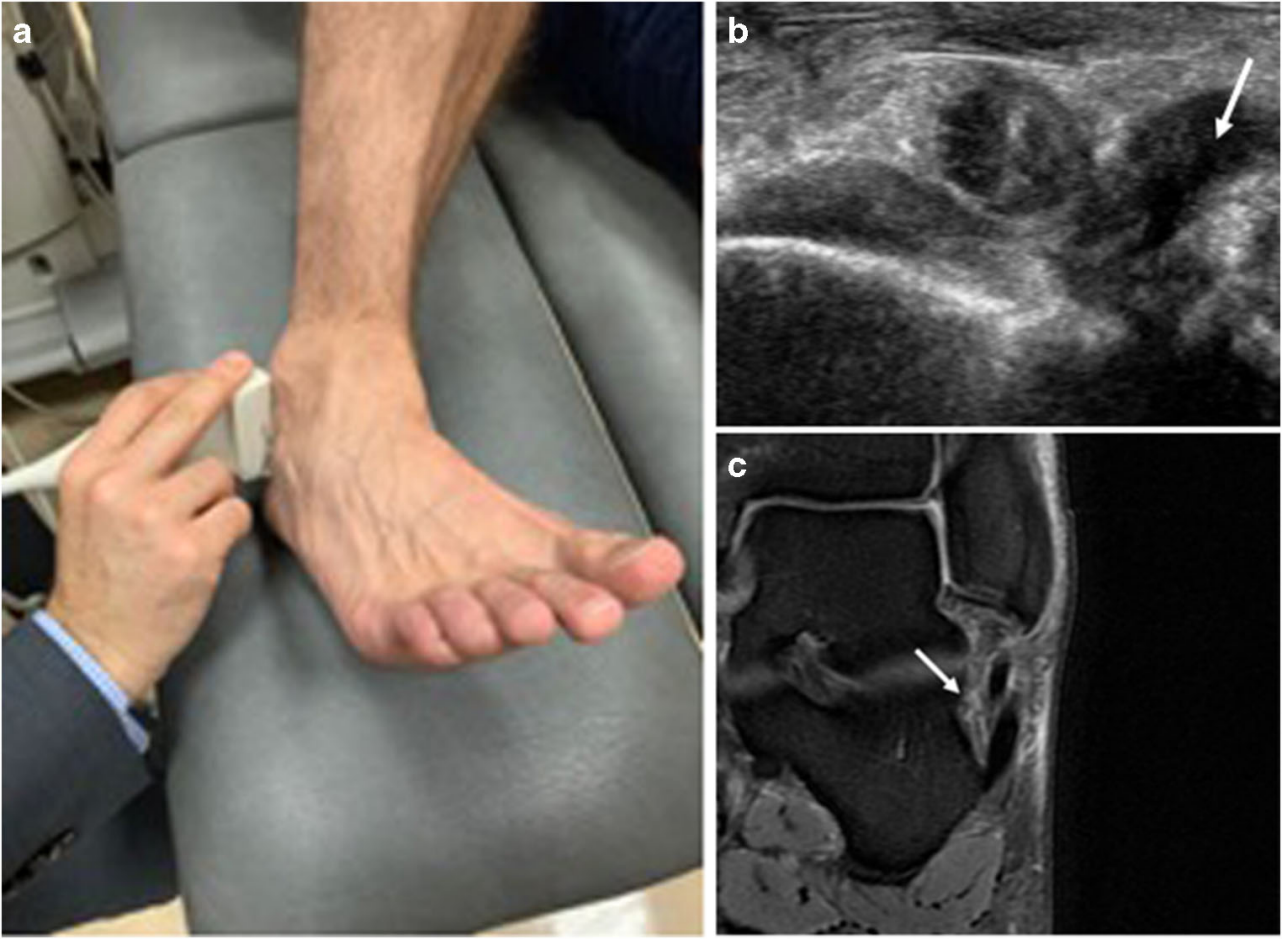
Probe position and (US/MRI) imaging results for the calcaneofibular ligament (CFL). Ultrasound for the diagnosis of calcaneofibular ligament (CFL) injury. a The ultrasound probe is placed in the frontal plane (longitudinal to the calcaneofibular ligament). b Ultrasound findings consistent with a complete tear of the calcaneofibular ligament (arrow). c Axial PD-FS image showing waviness of the calcaneofibular ligament consistent with a complete tear (arrow)
